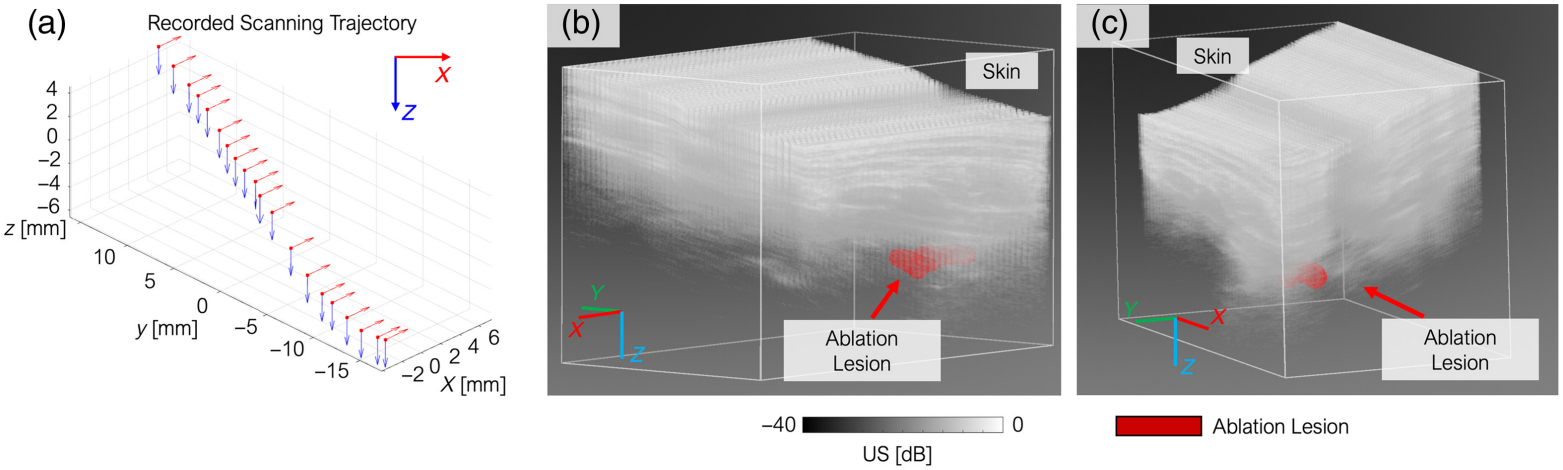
Reconstruction of post-ablation *in situ* liver lesion using robotic 3D scanning. (a) Trajectory of the ultrasound transducer along the abdominal skin surface recorded by the robotic system. (b), (c) 3D lesion maps reconstructed from photoacoustic imaging overlaid on anatomical structure, shown from two different viewing angles. Red voxels indicate the detected ablation-induced lesion, located in the shallow region of the liver beneath the skin.
